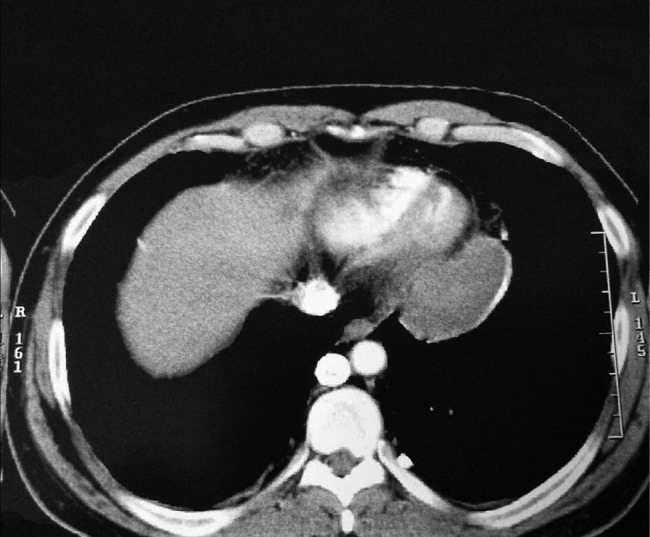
Chest CT with contrast showing mediastinal cysts.
